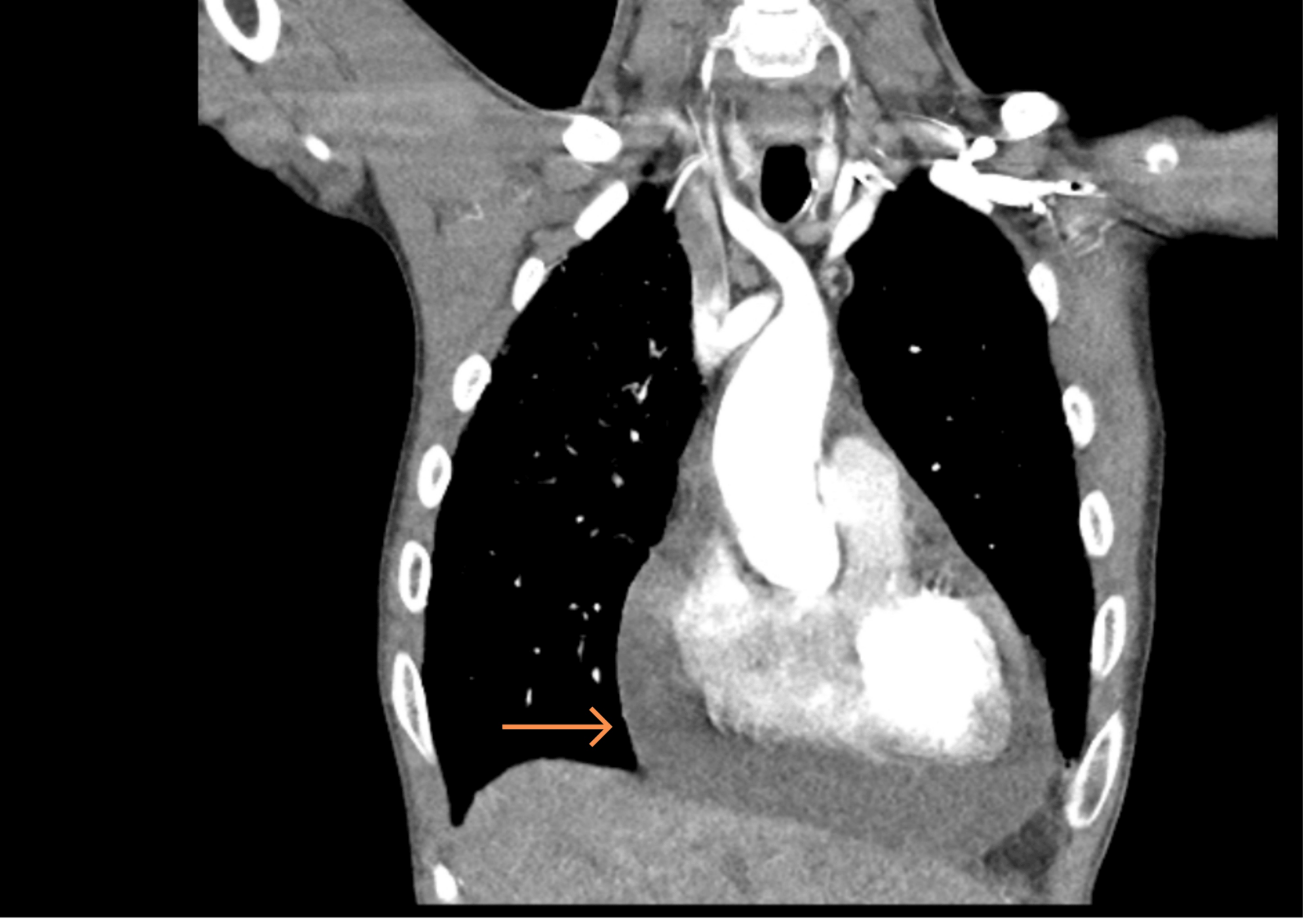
CTPA coronal view – moderate to gross volume pericardial effusion (orange arrow) CTPA: Computed tomography pulmonary angiogram
